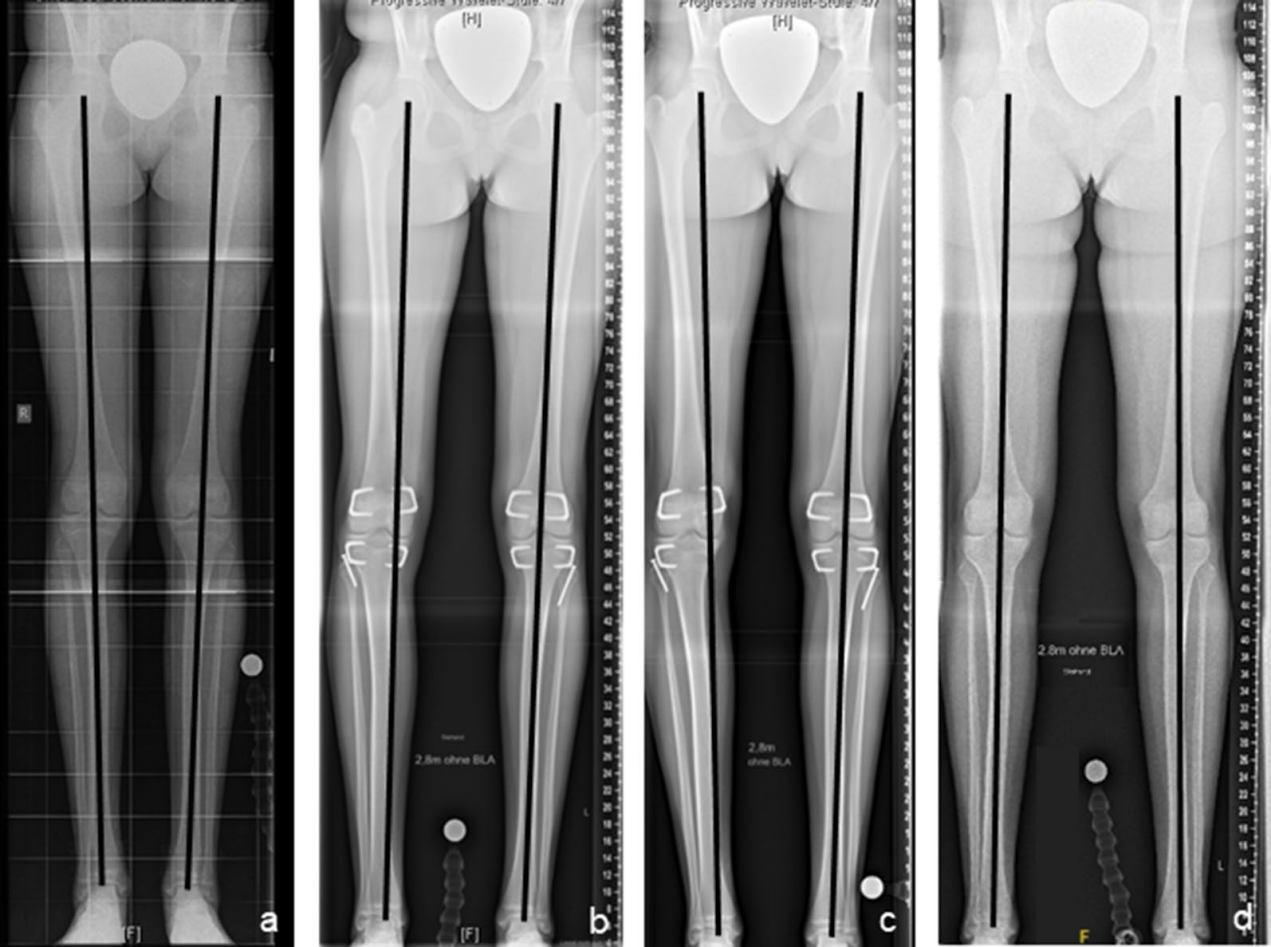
Secondary coronal malalignment after bilateral temporary epiphysiodesis using rigid staples (RigidTacks™) around the knee in a girl with idiopathic (familial) tall stature. Anteroposterior long standing radiographs right before surgery at the age of 10 years (a), after 2 years of temporary growth arrest, showing progressive iatrogenic tibial varus deformity on the right side (b), right after premature removal of the medial tibial rigid staple at the age of 12 years (c), and right after hardware removal at skeletal maturity (d), showing physiological coronal realignment and equal leg lengths
